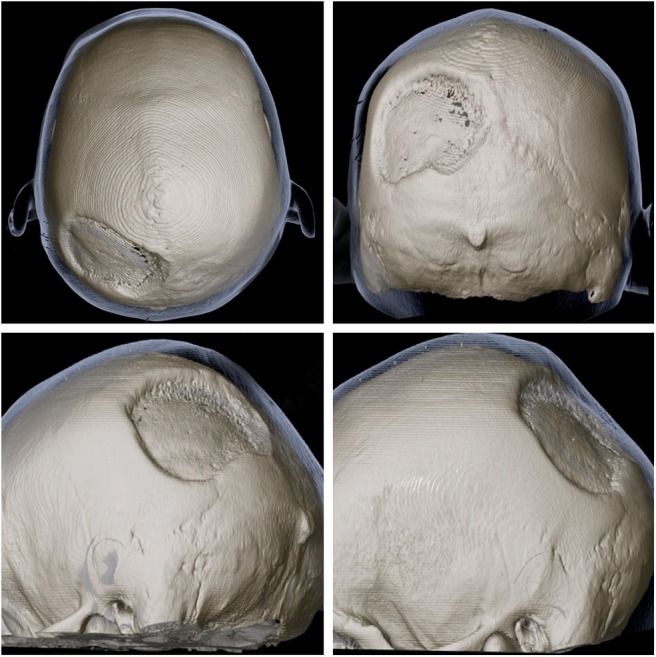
3D reconstruction of skull CT from multiple angles showing the extensiveness and location of the defect of the left parietal bone.
